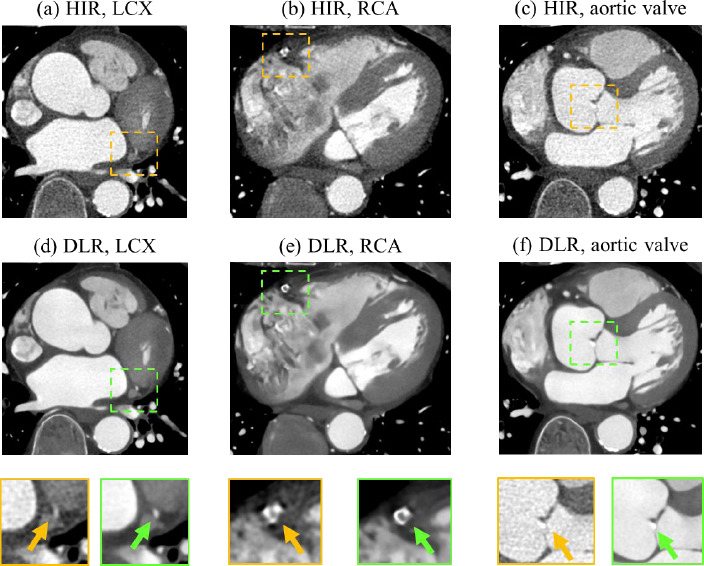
Coronary CTA images obtained in a 75-year-old woman with arrhythmia (average heart rate, 79 bpm). Images were acquired at a CTDI_vol_ of 17.8 mGy and reconstructed with HIR (a~c) and DLR (d~f). On DLR images, the distal segment of the Left Circumflex (LCX) is more conspicuous than on HIR images owing to the considerable reduction of image noise (a and d). The aortic valve structures, especially the leaflet, are more clearly visualized on DLR images, where the margin is blurred by the noise on HIR images. The calcified plaques in the Right Coronary Artery (RCA, b and e) and aortic valve (c and f) are more sharply delineated by DLR, contributing to the reduced partial volume and blooming artifacts.
